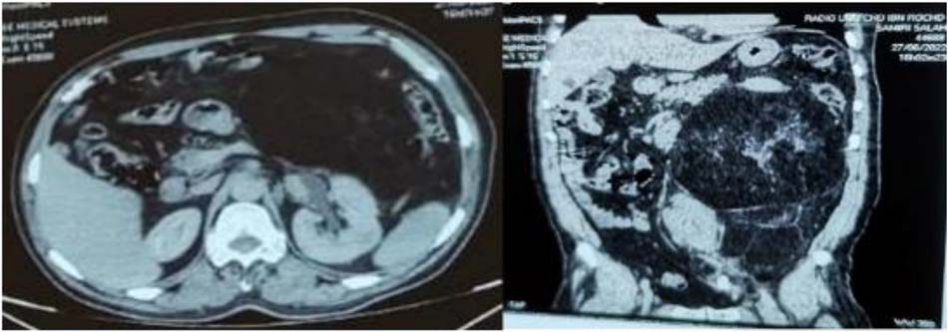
Axial and sagittal sections of an abdominal-pelvic CT scan showing a retroperitoneal liposarcoma compressing the surrounding structures and responsible for left pyelocaliceal dilation.
